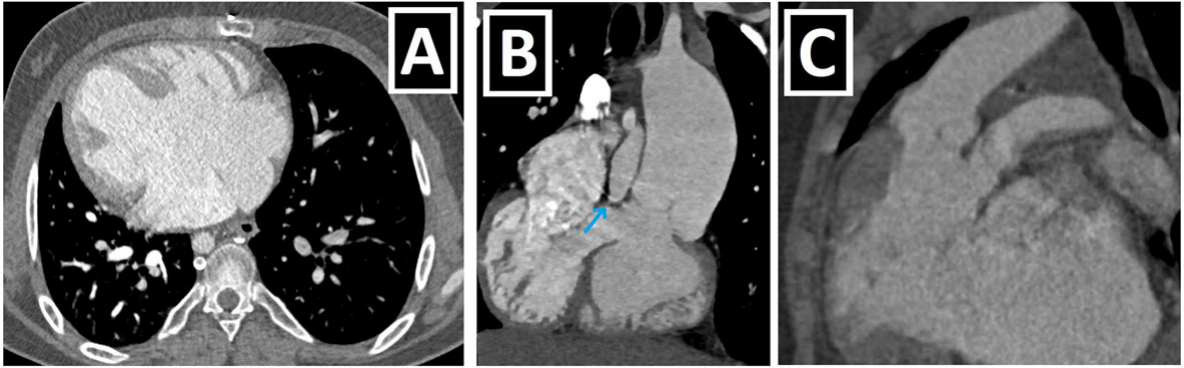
PLSVC with heterotaxy-3 (the most common accompanying cardiac anomalies). Axial (a), coronal-oblique (b), and sagittal-oblique reformatted (c) CT images depict the three most common cardiac anomaly seen in the PLSVC and heterotaxy coexistence: AVSD (with dextrocardia) (a), pulmonary atresia (with VSD) (blue arrow, b) and DORV (c)
